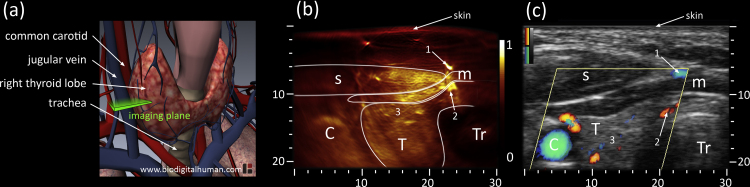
(a) Anatomy of thyroid gland including cardio-vascular and respiratory system; the cross-sectional 2D imaging plane is highlighted in green. (b) Optoacoustic and (c) ultrasound cross-sections of the left thyroid lobe of the first volunteer. The optoacoustic image, leveled and normalized from 0 to 1, shows with high sensitivity vascular features of skin, muscles [1], [2] and within the thyroid lobe [3]. The corresponding ultrasound image in grayscale also depicts Directional Power Doppler signals superimposed in color (red/blue colormaps indicating opposite flow directions). C: Carotid, T: Thyroid, Tr: Trachea, s: sternocleidomastoid muscle, m: infrahyoid muscle; axes in mm.
